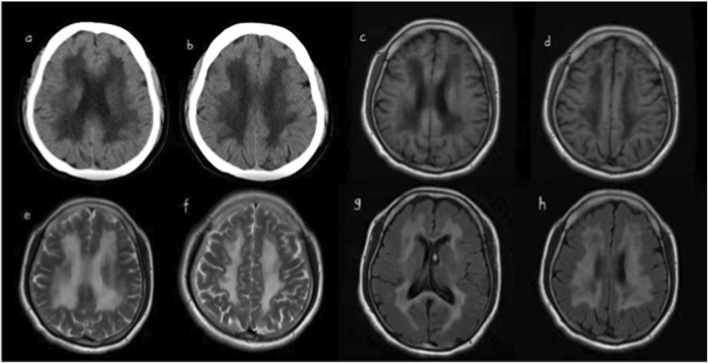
(a, b) are cranial CT scans, showing demyelinating changes in the periventricular white matter and white matter of the semioval center bilaterally. Hypointense signals are visible in the anterior and posterior horns of the lateral ventricles on T1-weighted images (c,d). Slightly hyperintense signals are displayed in the anterior and posterior horns of the lateral ventricles on T2-weighted images (e,f). Extensive demyelinating changes in the periventricular white matter and white matter of the semioval center can be observed on T2-FLAIR images (g,h).
